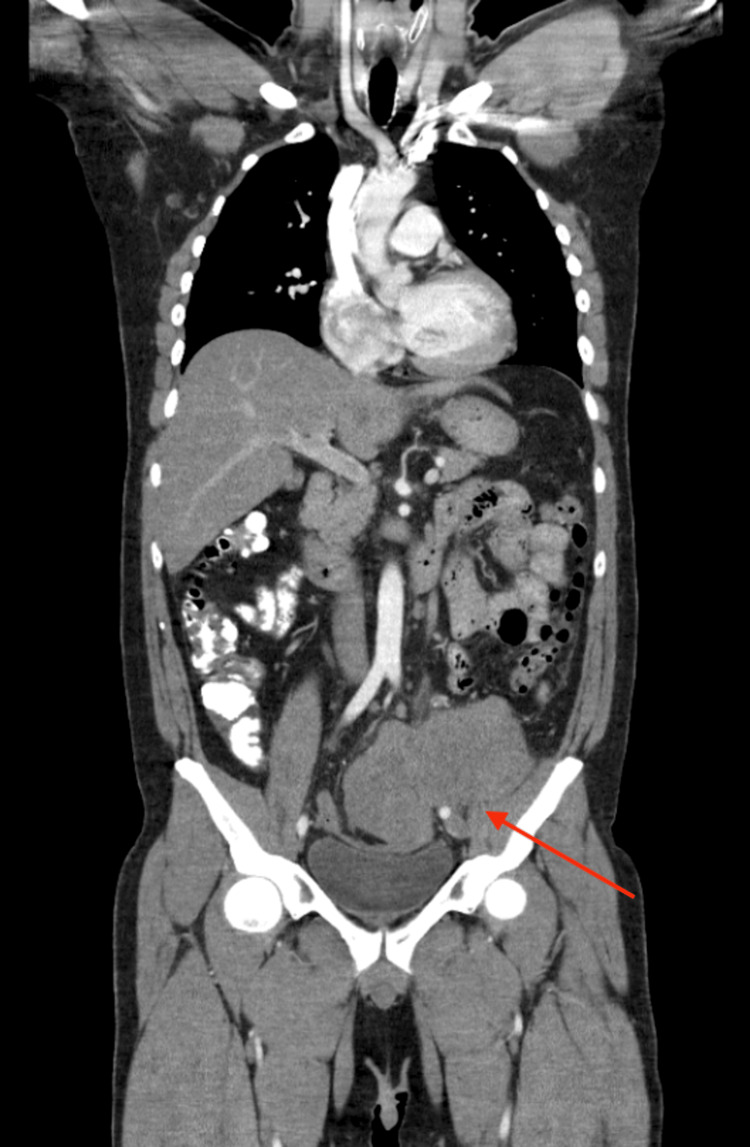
Computed tomography (CT) scan of the chest, abdomen, and pelvis showing a lobulated heterogeneous soft tissue mass in the left lower quadrant in the abdomen, measuring approximately 10 x 13 cm (red arrow])
